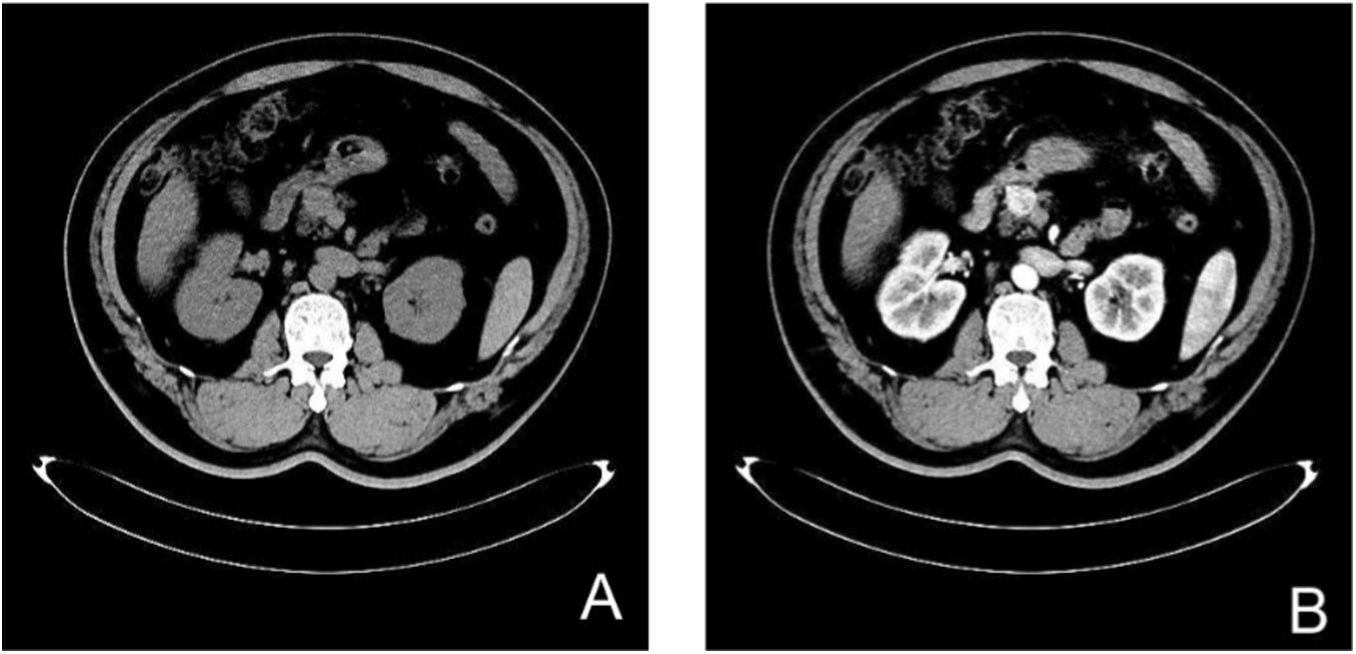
Imaging examination of insulinoma. A CT indicated a 2 × 3 cm occupancy at the junction of the pancreatic head and neck; (B) the occupancy with vascularity in CT enhanced scan
